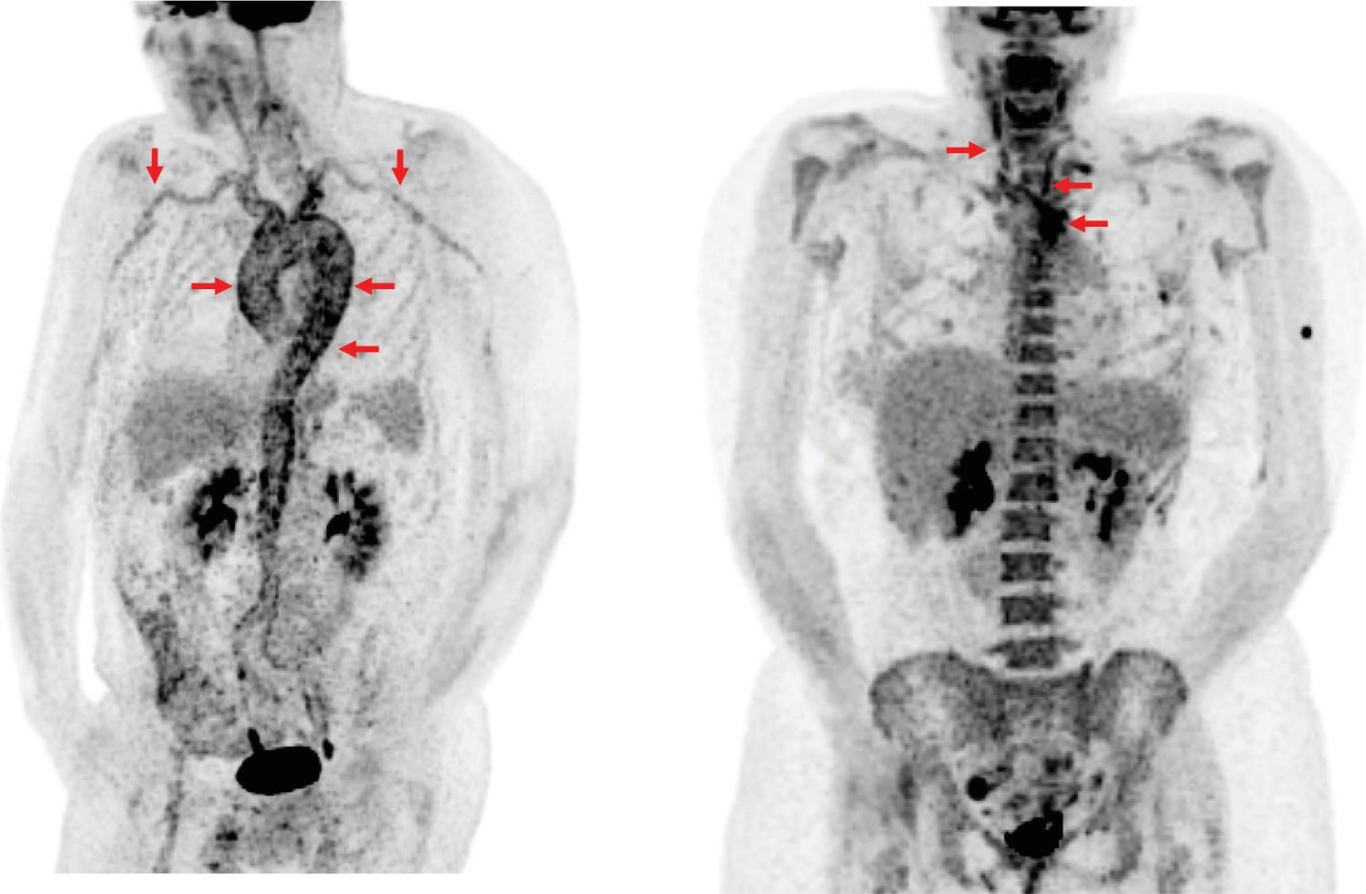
Representative FDG-PET scan images from a patient with giant cell arteritis (GCA) and a Patient with Takayasu’s Arteritis (TAK). A representative FDG-PET scan from a patient with newly diagnosed GCA is depicted (left image). The patient has severe vascular FDG uptake (radiotracer in black) diffusely throughout the aorta and bilateral subclavian/axillary arteries (red arrows). This pattern of diffuse vascular FDG uptake throughout the aorta is characteristic for GCA. A representative FDG-PET scan from a patient with newly diagnosed TAK is also shown (right image). The patient has severe vascular FDG uptake (black) in the aortic arch and bilateral carotid arteries. This focal vascular uptake throughout specific arterial territories is characteristic for TAK. *Images courtesy of the NIAMS vasculitis translational research program*
